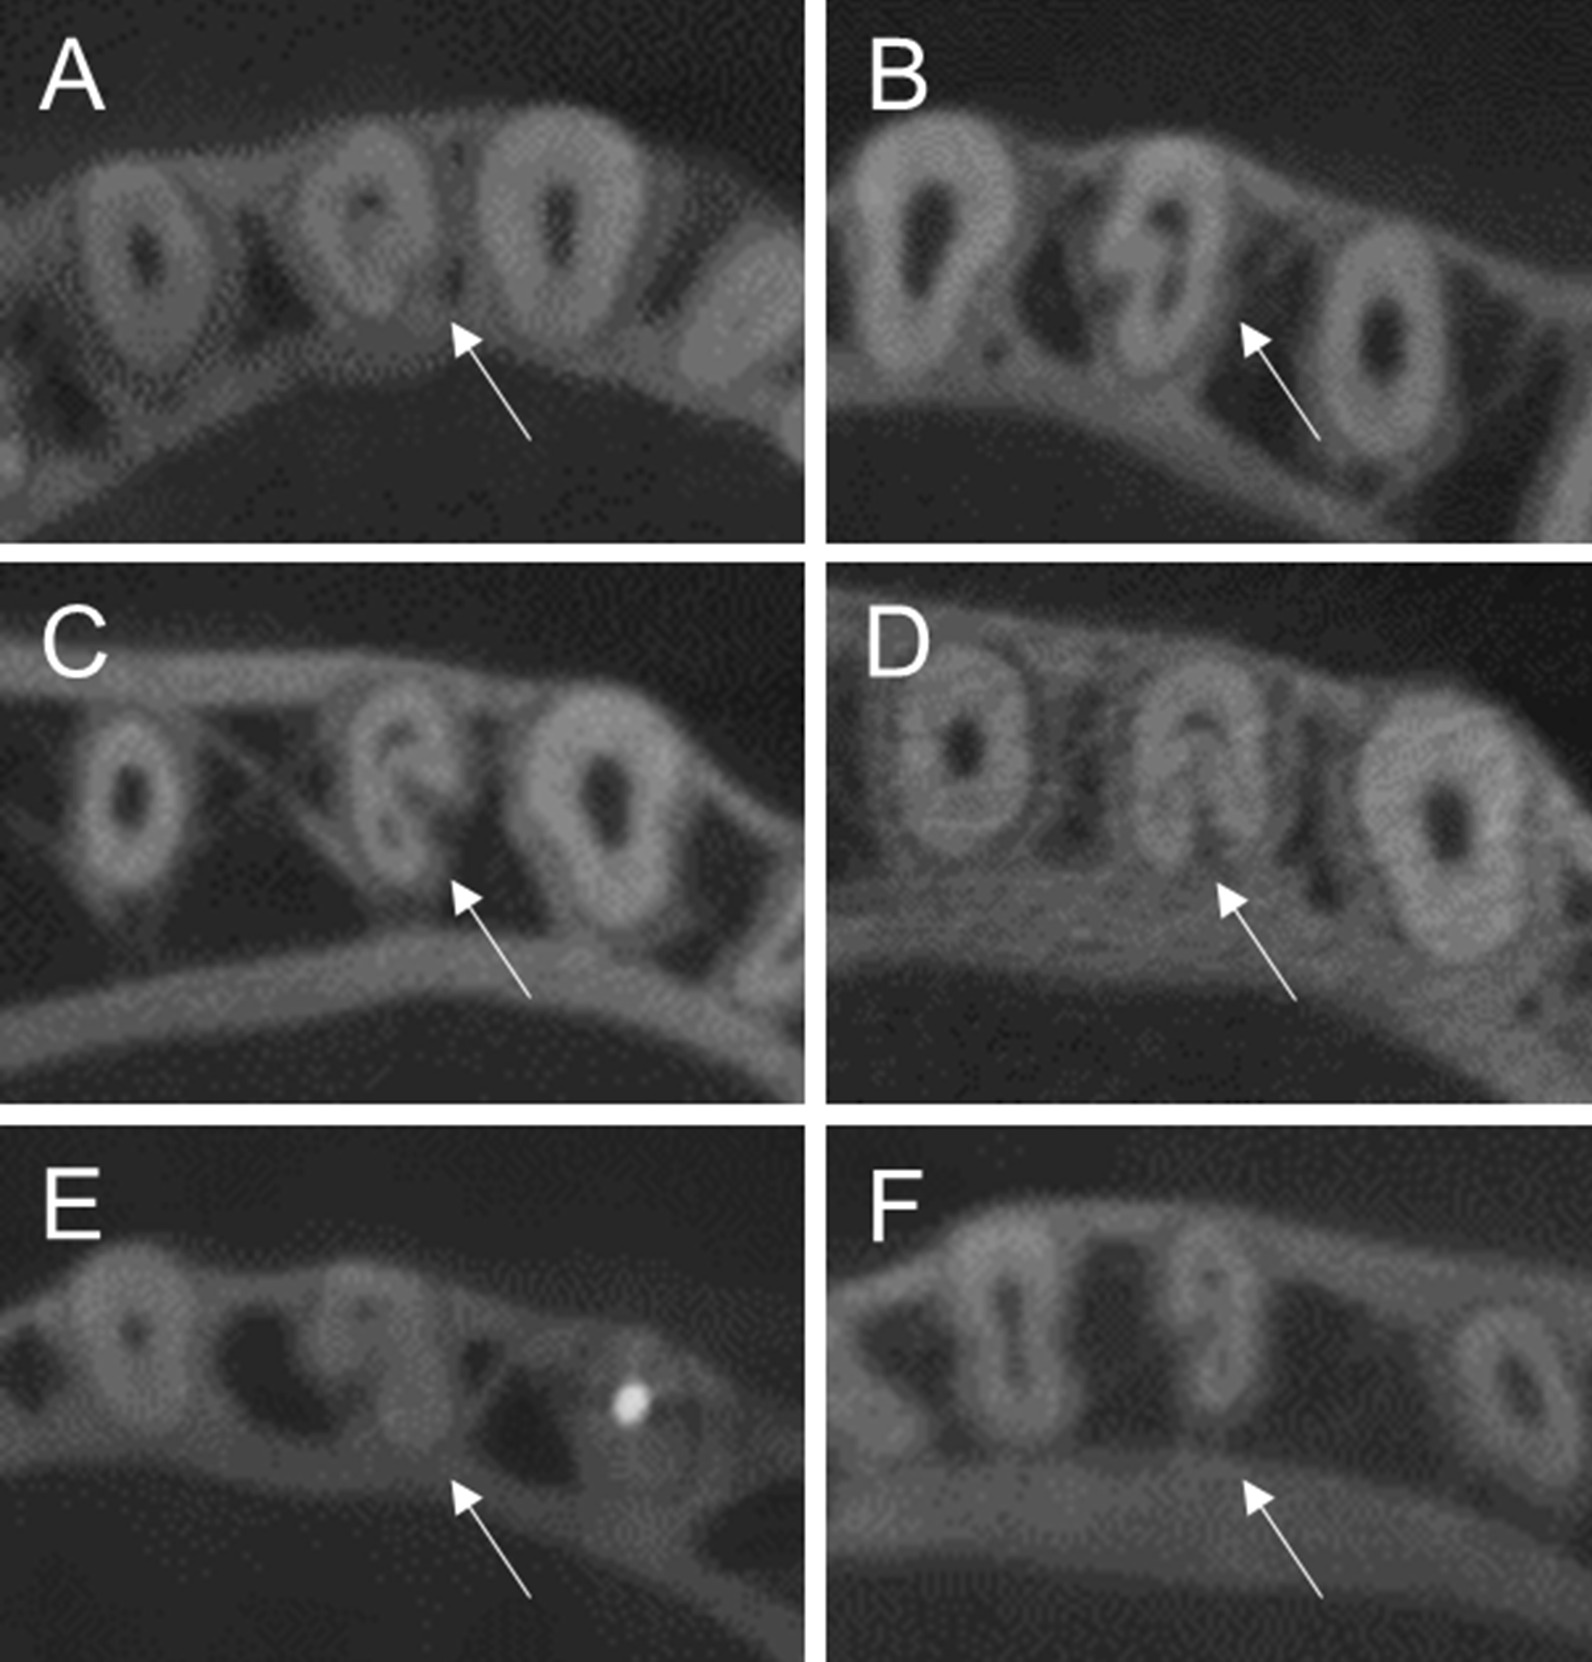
Axial views of CBCT images demonstrate C-shaped root canals in mandibular premolars A, B Category I, C, D Category II, E, F Category III. (Arrows indicate C-shaped root canals in mandibular premolars)
